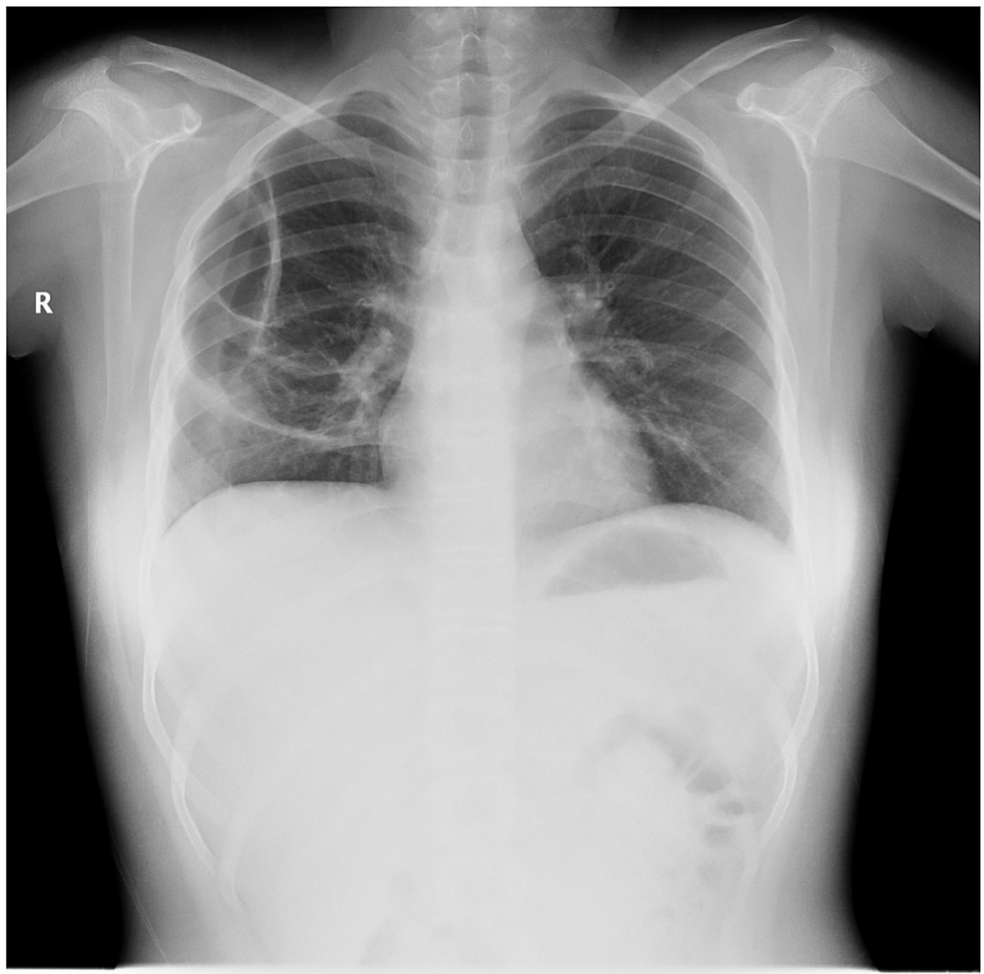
Chest radiograph (posteroanterior view). This radiograph, obtained after six weeks, shows marked improvement with remaining large pneumatoceles.
